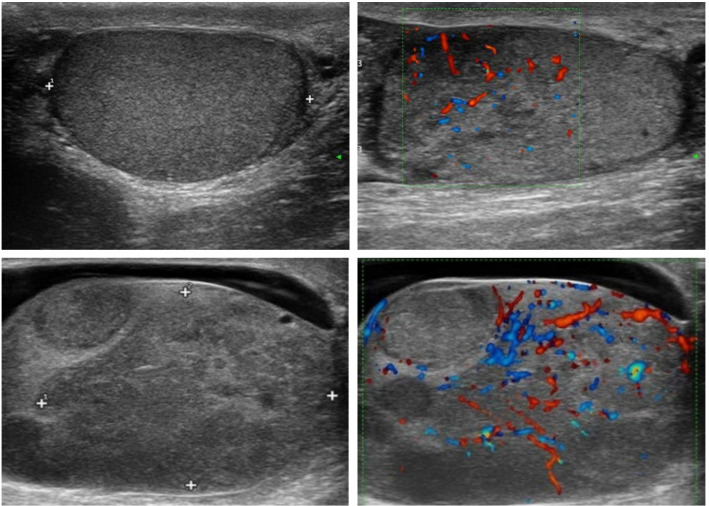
Testicular ultrasound and color Doppler flow imaging findings. Ultrasound and color Doppler flow imaging (CDFI) images are shown in a 2 × 2 panel, arranged from left to right and top to bottom. Ultrasound and color Doppler flow imaging (CDFI) of the testis at the patient’s initial visit. Follow-up ultrasound and CDFI images showing heterogeneous internal echogenicity with multiple hypoechoic areas, the largest measuring approximately 39 × 30 mm, with abundant internal blood flow signals. (2×2 grid): A (top-left), B (top-right), C (bottom-left), D (bottom-right).
